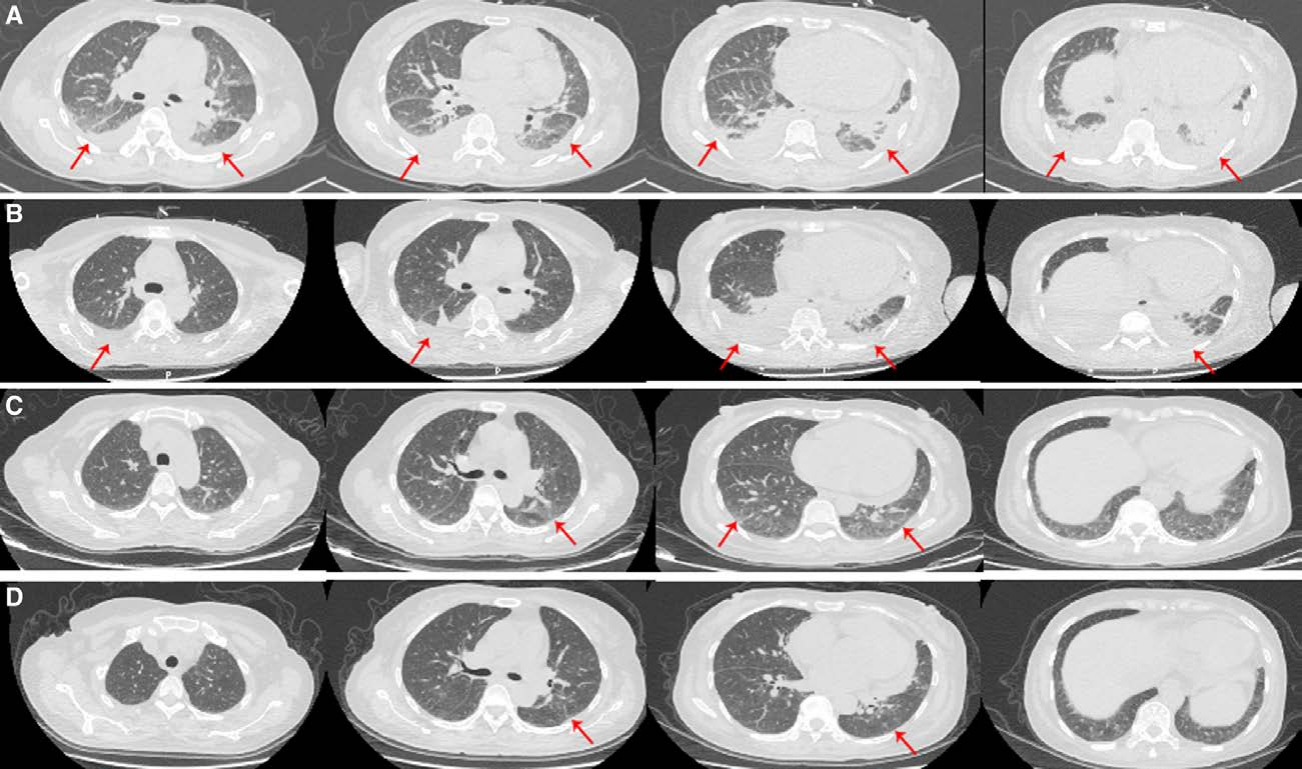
Chest CT images of the patient at later stages of disease. (A) CT image of the patient on November 5, arrows showed a large amount of ground-glass lesions and moderate amount of pleural effusion in both lungs. (B) CT image of patient on November 8, arrows showed a large amount of ground-glass lesions and moderate amount of pleural effusion slightly reduced than before. (C) CT image of patient on November 14, arrows showed significantly less ground-glass lesions in both lungs, and pleural effusion had been absorbed. (D) CT image showed only a small amount of inflammatory lesions in both lungs when the patient came back to hospital for reexamination 1 mo after discharge.
